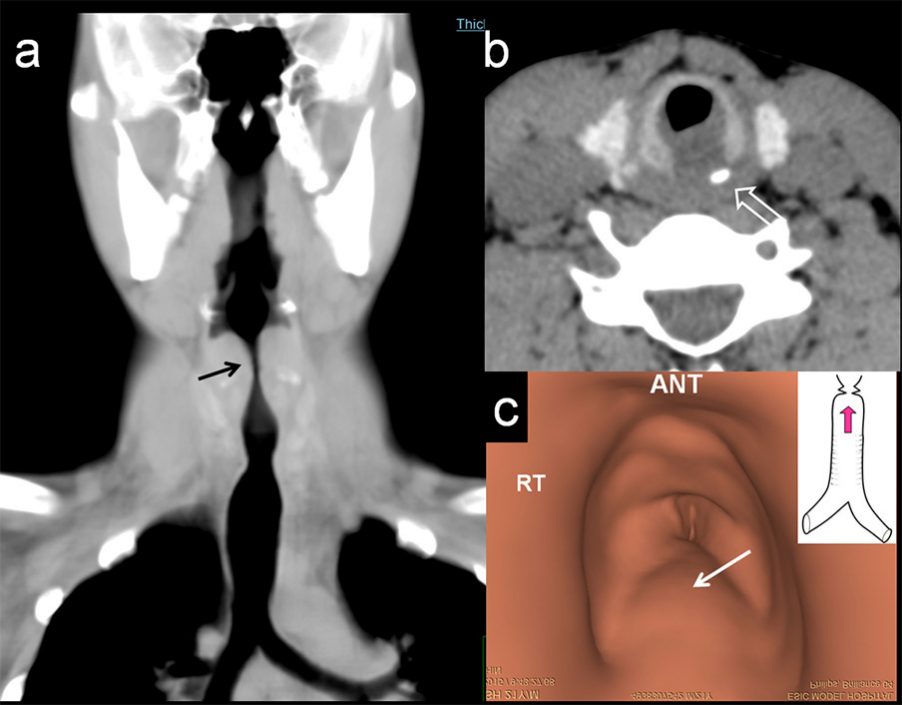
Coronal minimal intensity projection (a) shows a diffuse soft tissue swelling (black arrow) centred around the posterior arch of the cricoid cartilage with reduction in the airway calibre. (b) Axial CT image showing the cricoid bone fragment with the soft tissue swelling (open arrow). (c) Endoscopic view of the subglottic region from volumetric CT data, viewed caudocranially towards the subglottic region (inset) shows diffuse soft tissue swelling along the posterior wall of the subglottic trachea (arrow).
